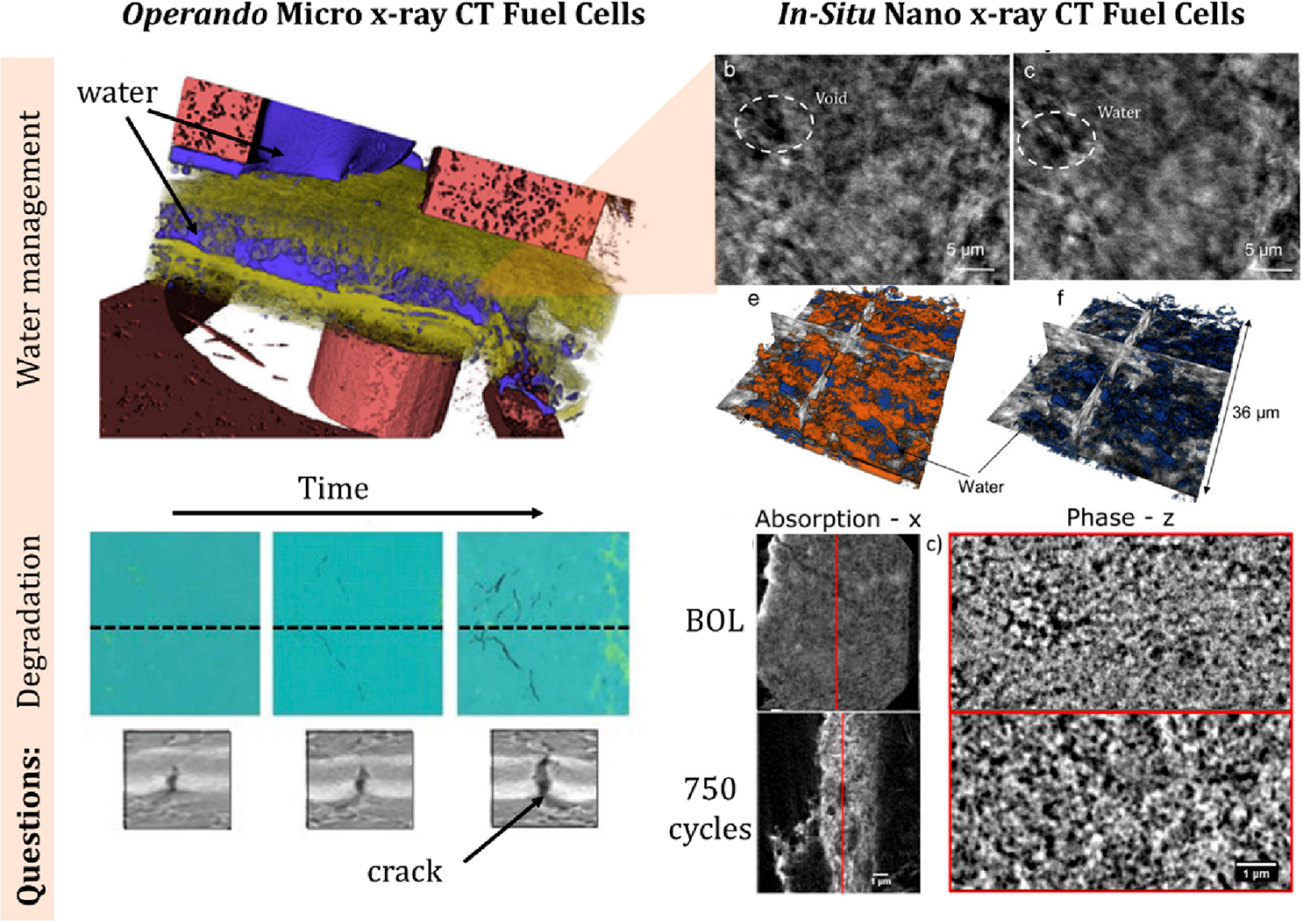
Examples of operando micro-X-ray CT studies and in situ nano-X-ray
